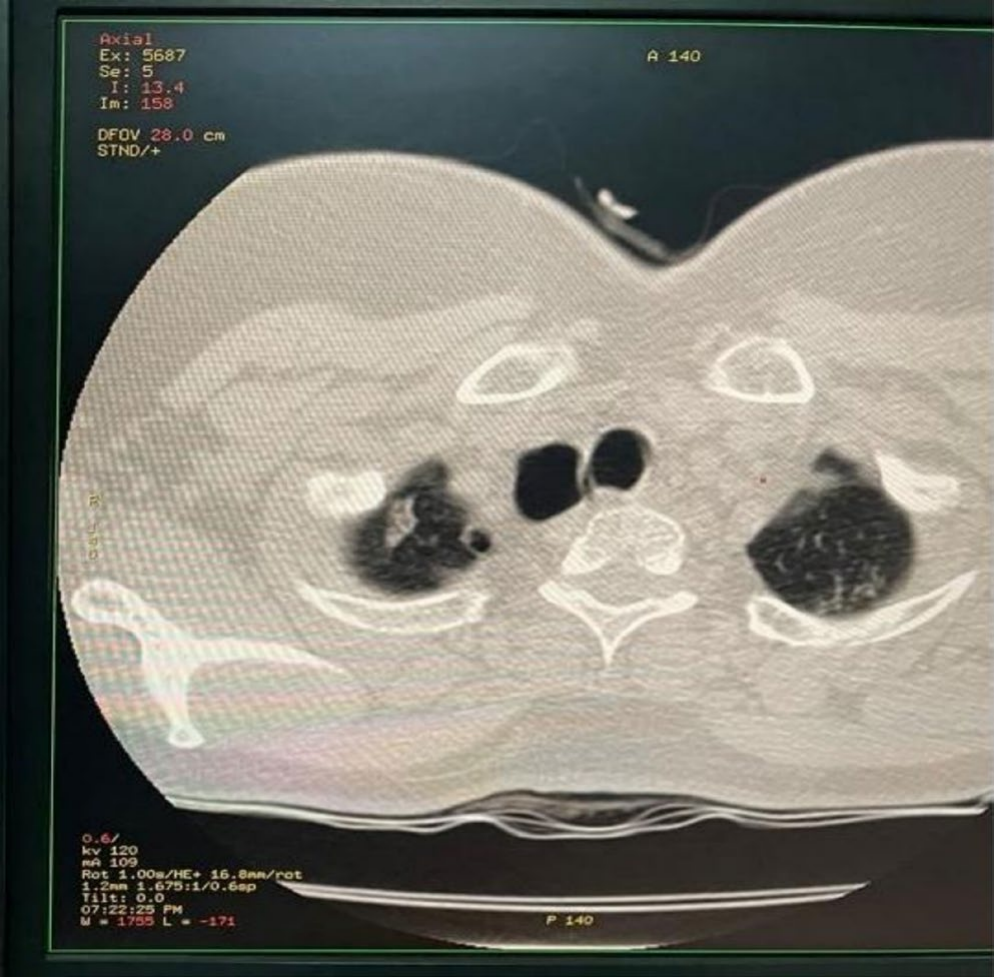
Contrast‐enhanced computed tomography scan of the neck shows a 4 × 5 cm tracheal diverticulum. It is arising from the right posterolateral tracheal wall at the level of the fifth to seventh tracheal rings. The air‐filled outpouching demonstrates clear communication with the tracheal lumen.
